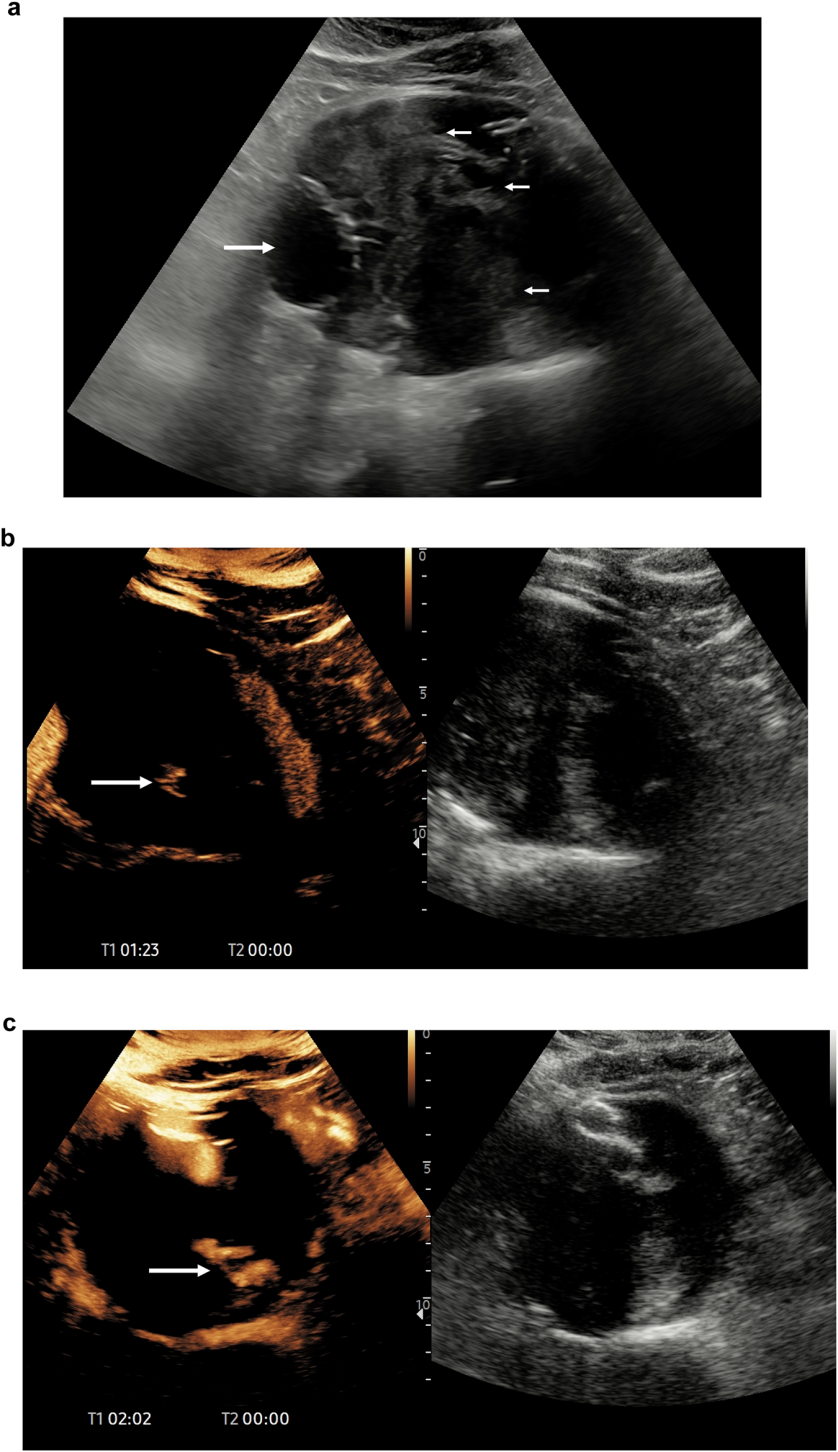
An 81-year-old man underwent an EVAR three years prior, with repeated CT examinations showing a persistent sac dilatation and no endoleaks. The patient was referred for a CEUS examination. a The baseline B-mode ultrasound examination demonstrates the aortic stent graft (arrow) with mixed echogenic contents of the aortic sac (small arrows), suggesting evolving haematoma. b Following the administration of 1.2 mL of SonoVue™ (Bracco SpA, Milan), at 1 min 23 s post-administration, there is an endoleak demonstrated (arrow). c At 2 min 2 s, on a maximum intensity projection, there is accumulation of contrast (arrow) at the site of the endoleaks from posterior lumbar vessels. The CECT examinations did not show the late endoleaks, whereas the real-time continuous examination with ultrasound enabled a longer examination to demonstrate the endoleak
